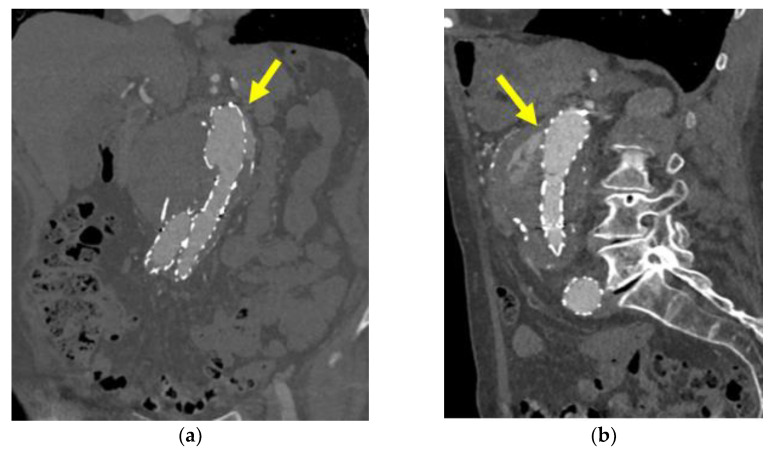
CTA demonstrating a type Ia endoleak after infrarenal endovascular aneurysm repair for AAA: (a) coronal and (b) sagittal views demonstrate the contrast flow (yellow arrow) within the aneurysm sac entering from the proximal end of the stent-graft; CTA, computed tomography angiography; AAA, abdominal aortic aneurysm.
